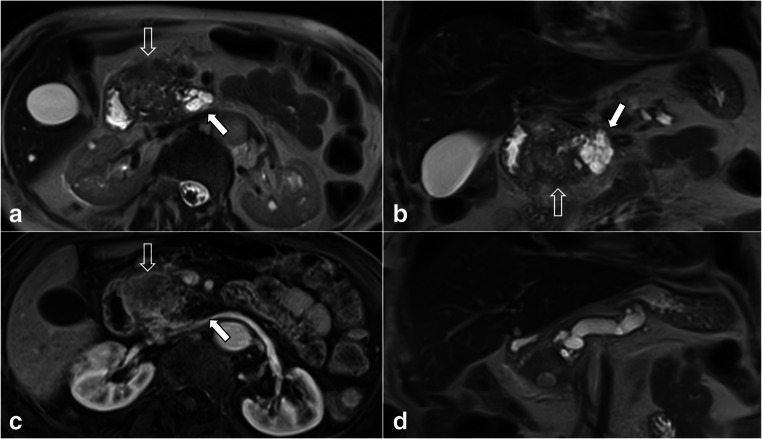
Pancreatic MRI of an 80-year-old patient with weight loss and abdominal pain. The axial (a) and coronal (b) T2-weighted images show a solid-mass-forming pancreatic cancer (PC) (open arrows) originating from an adjacent IPMN (white arrows) located in the head of the pancreas. The pancreatic cancer is homogeneously hypointense in the T1-w axial image in the pancreatic arterial phase (c). The mass-forming PC causes a stricture of the main pancreatic duct (MPD) with upstream dilatation on coronal T2-weighted image (d). The patient was excluded from our cohort, as the dilation of the MPD upstream secondary to a solid mass may lead to overestimation of the positive yield of the finding “dilated MPD”
